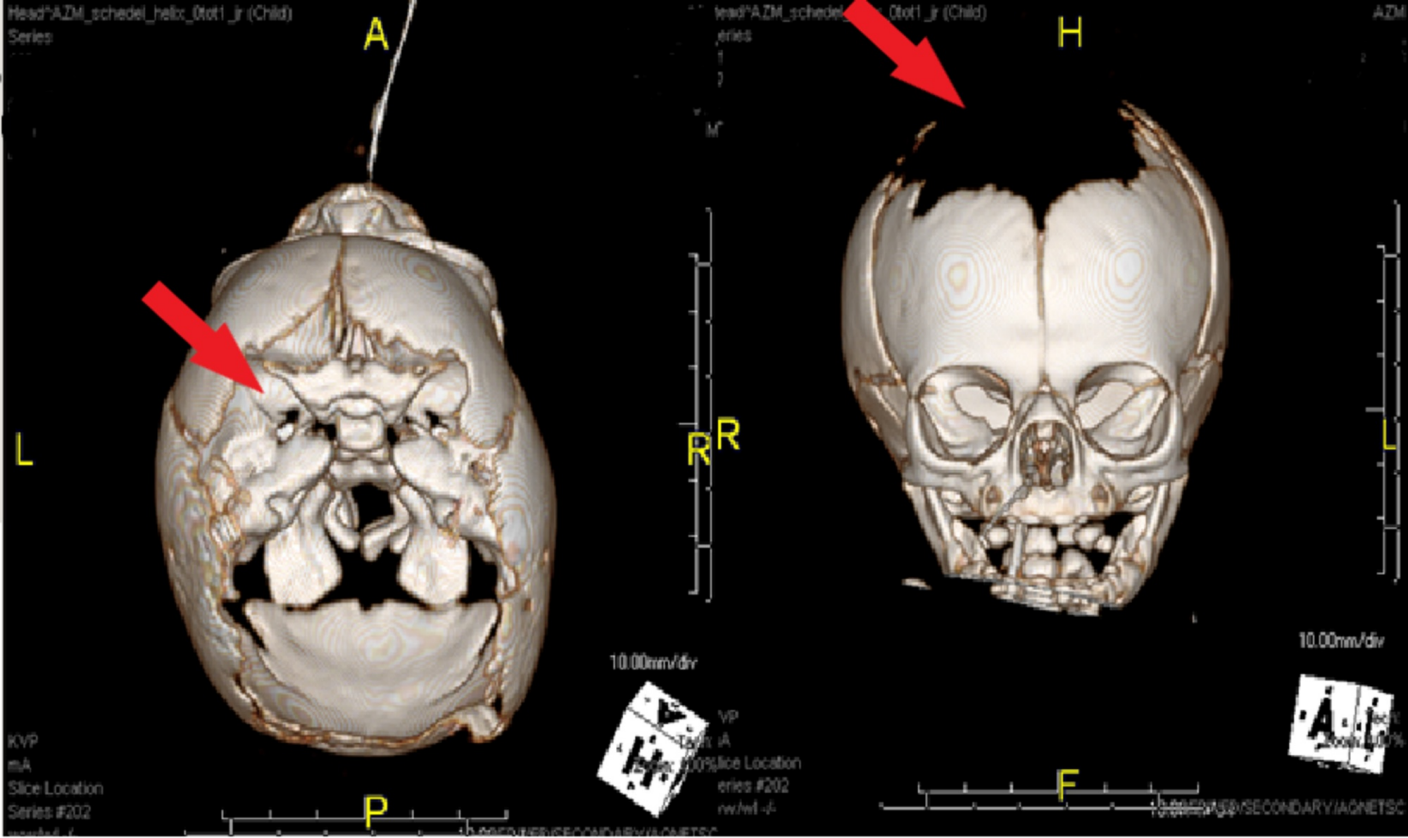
3D representation of postnatal computed tomography scan which verified the extensive defect of the scalp and skull Left image: cranial view. Right image: frontal view.
